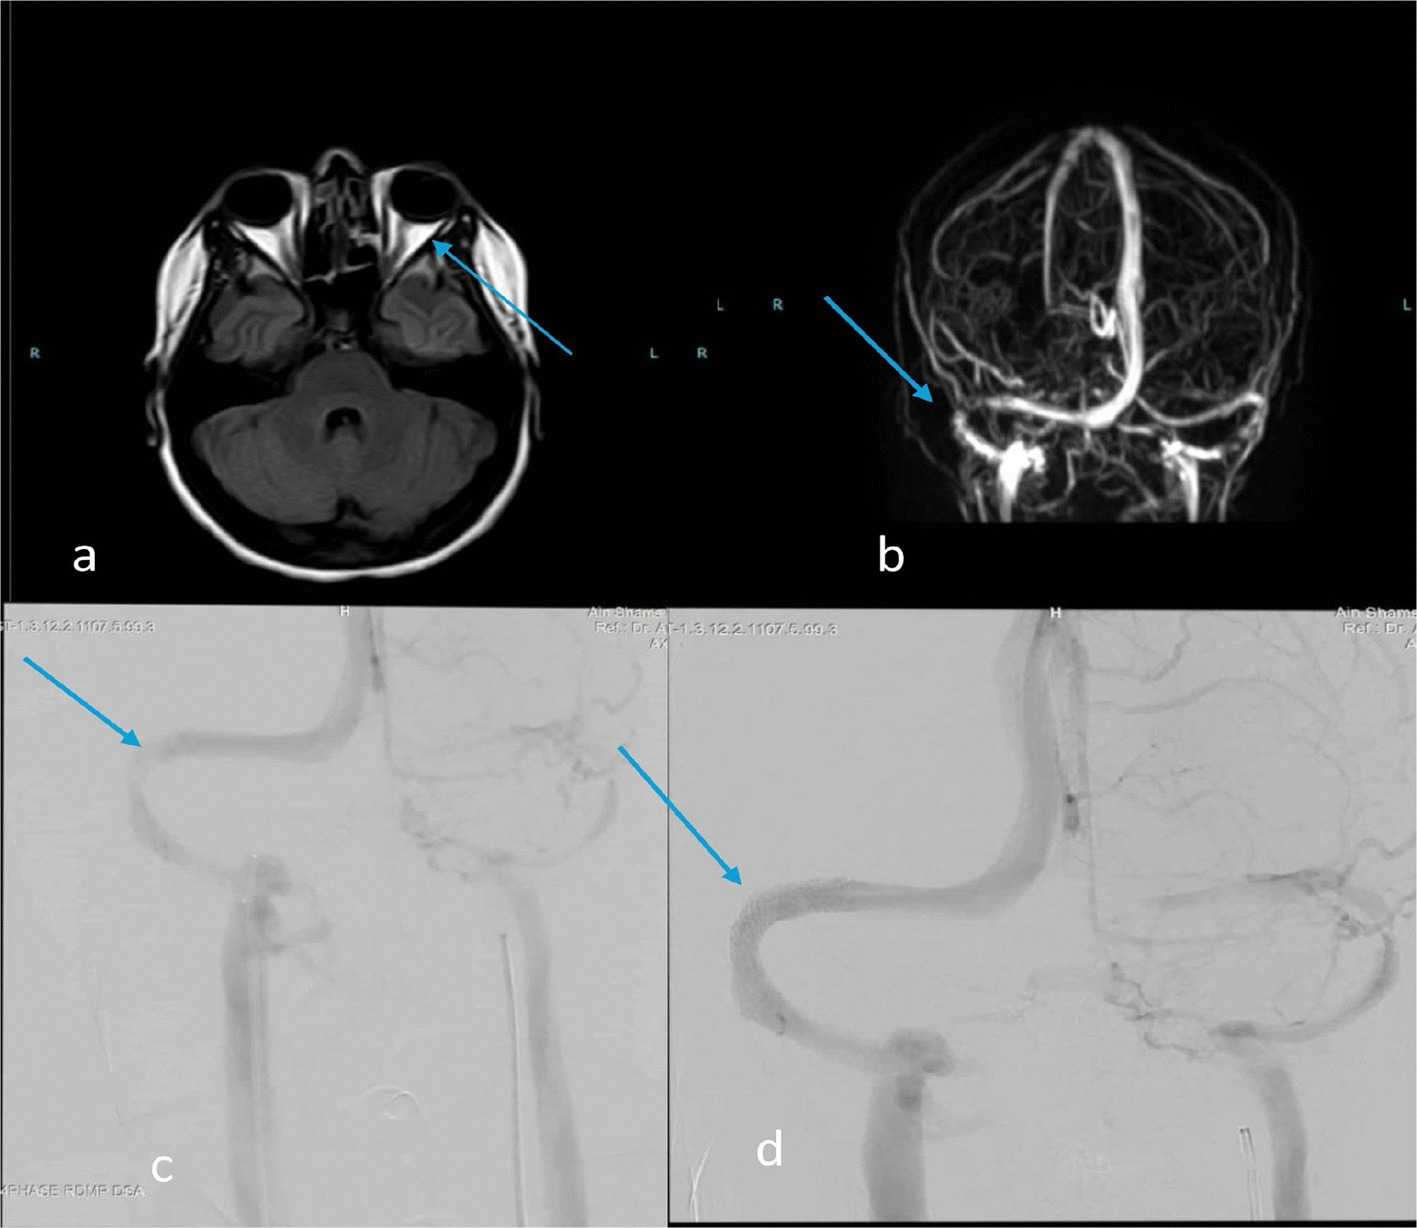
a axial T1 weighted brain MRI showing distention of optic nerve sheath complex. b MR venography demonstrating focal stenosis of right transverse sigmoid sinus junction. c Digital subtraction venography confirming significant stenosis. d Post-stenting DSV image showing restoration of normal venous outflow across the previously stenotic segment
